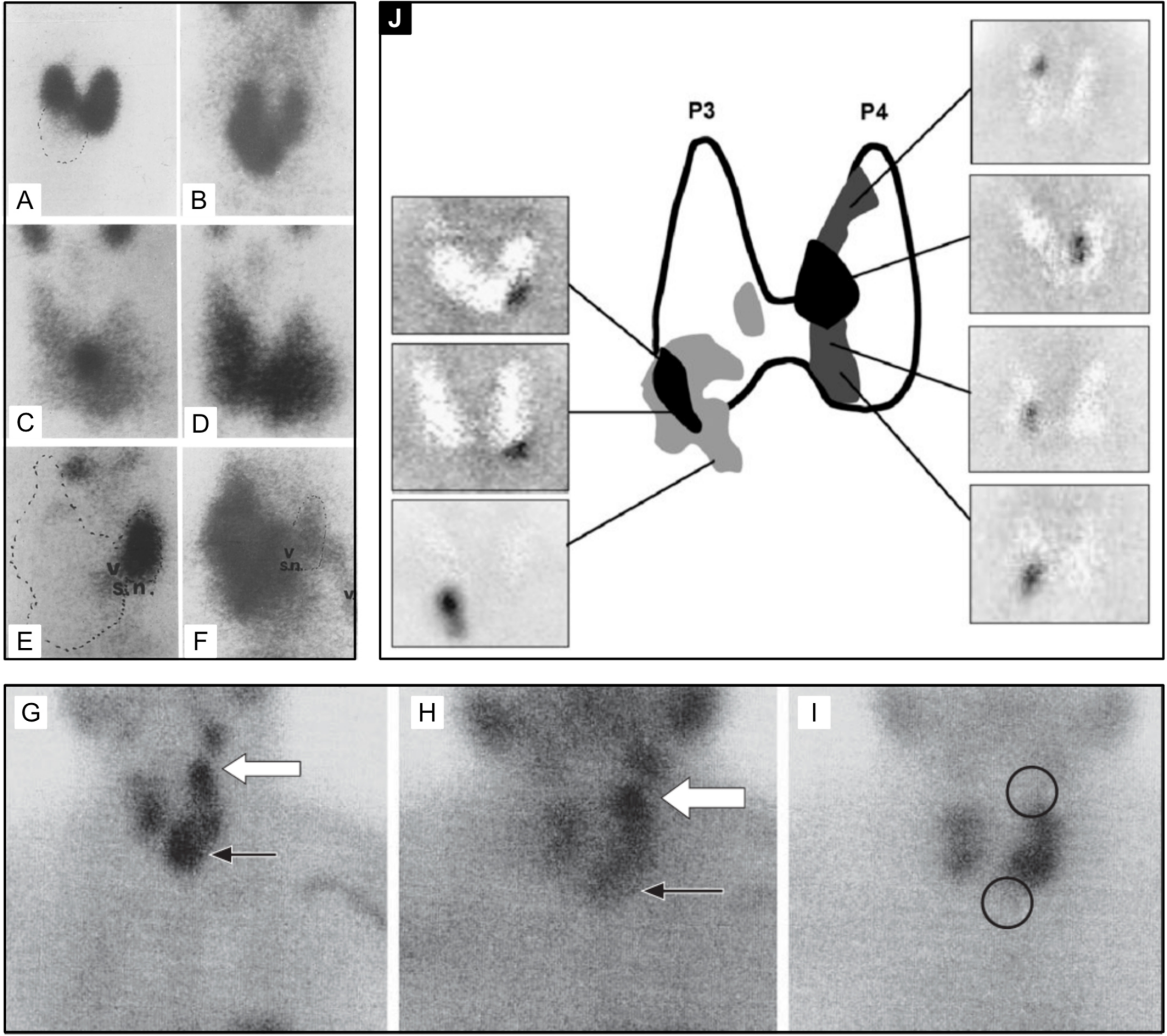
99mTc-sestamibi with or without 99mTc-pertechnetate imaging is the current standard of care for PA detection at most institutions at the time of writing of this article. Thyroid nodules may confound parathyroid imaging using this method as they have variable uptake relative to the thyroid gland with 99mTc-pertechnetate (A, C, E, I) and with 99mTc-sestamibi (B, D, F, G, H). Although benign thyroid nodules typically washout on delayed 99mTc-sestamibi imaging (H), thyroid carcinoma [(G, H), white arrow] can have retained uptake similar to parathyroid adenoma [(G, H), black arrow]. Panel (J) provides an atlas of PA appearances on dual tracer 99mTc-sestamibi minus 99mTc-pertechnetate subtraction and the frequency of PA locations relative to the thyroid gland (highest frequency-black, lower frequency-grey). Superior PA are derived from the fourth pharyngeal pouch (P4) and therefore more posterior. Inferior PA are derived from the third pharyngeal pouch and therefore more anterior (P3). SPECT imaging can aid in distinguishing the anterior versus posterior location. Reproduced with permission from: (A–F) Földes I, Lévay A, Stotz G. Comparative scanning of thyroid nodules with technetium-99m pertechnetate and technetium-99m methoxyisobutylisonitrile. Eur J Nucl Med. 1993;20: 330–333. doi: 10.1007/BF00169809. (G–I) Lorberboym M, Ezri T, Schachter PP. Preoperative technetium Tc 99m sestamibi SPECT imaging in the management of primary hyperparathyroidism in patients with concomitant multinodular goiter. Arch Surg. 2005;140: 656–660. doi: 10.1001/archsurg.140.7.656. (J) Taïeb D, Hassad R, Sebag F, Colavolpe C, Guedj E, Hindié E, et al. Tomoscintigraphy improves the determination of the embryologic origin of parathyroid adenomas, especially in apparently inferior glands: imaging features and surgical implications. J Nucl Med Technol. 2007;35: 135–139. doi: 10.2967/jnmt.107.039743.
